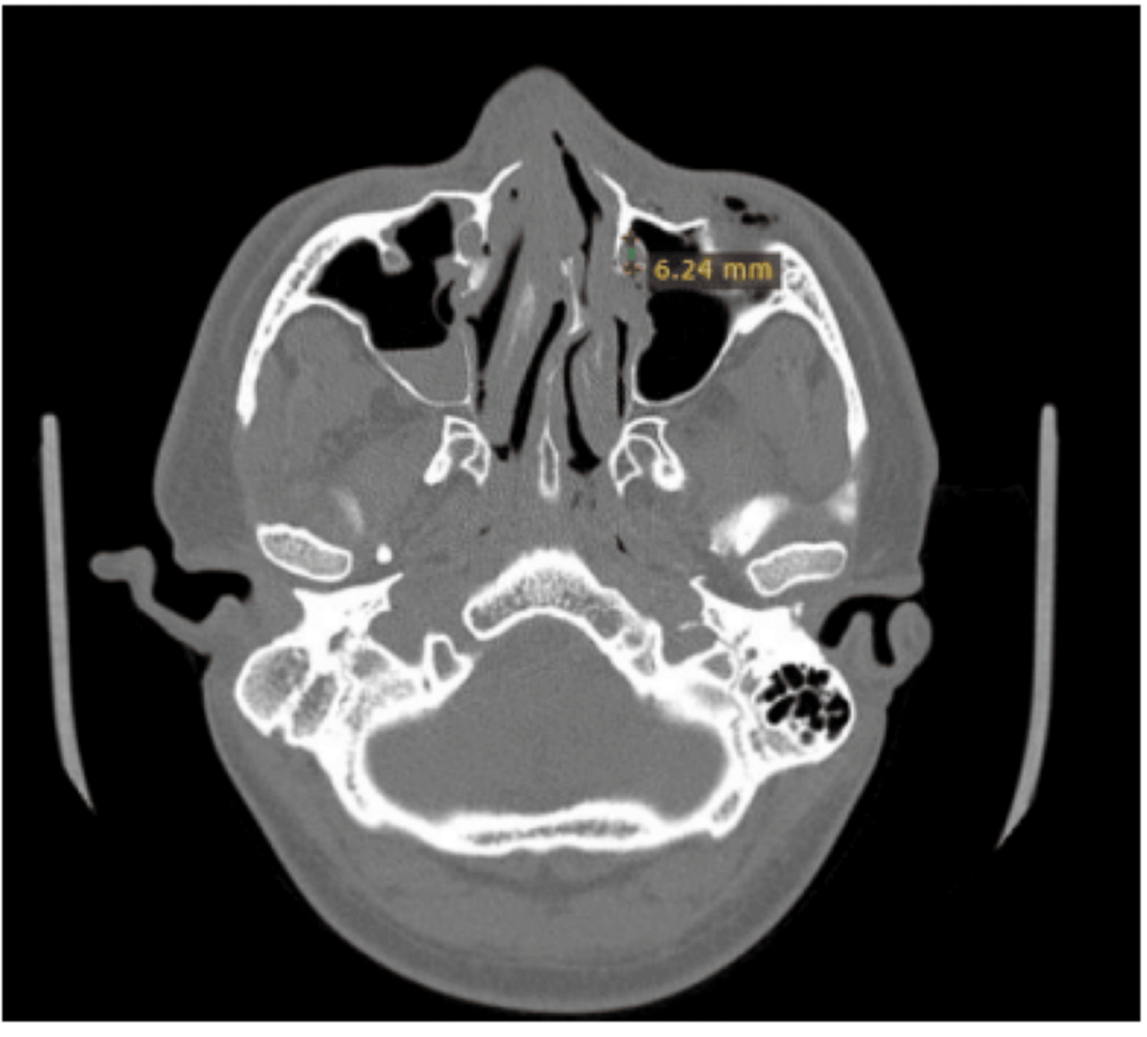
The axial section of the computed tomography scan of the fronto-naso-orbito-ethmoid fracture shows calibrated measurements using advanced tools to measure the collapse in the bony nasolacrimal canal on the radiographically assessed fracture site.
